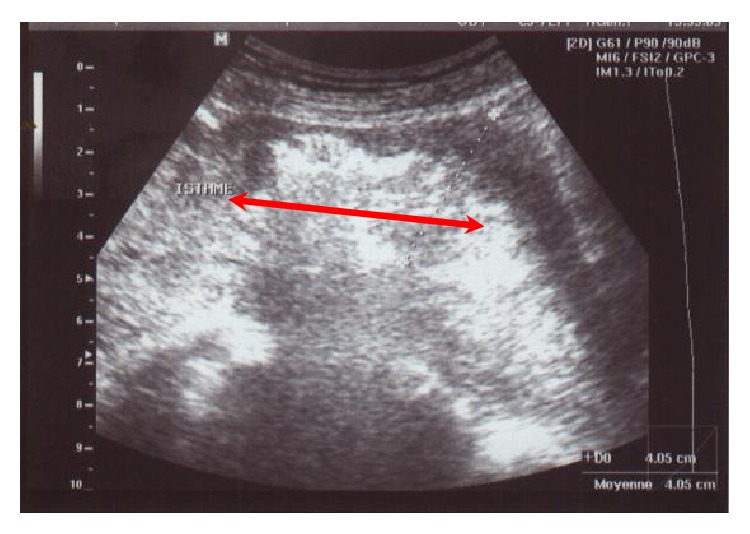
Ultrasonography aspect of the placenta increta fragment.
